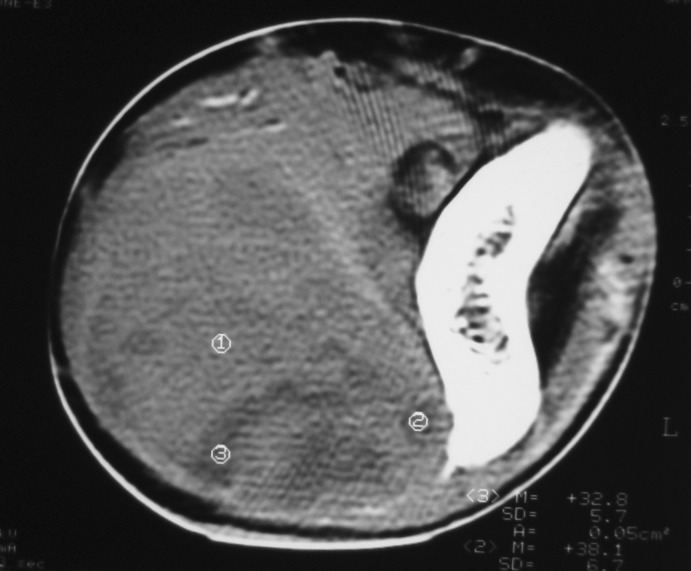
Unenhanced computed tomography image showing a solid soft tissue tumor with necrotic components.
